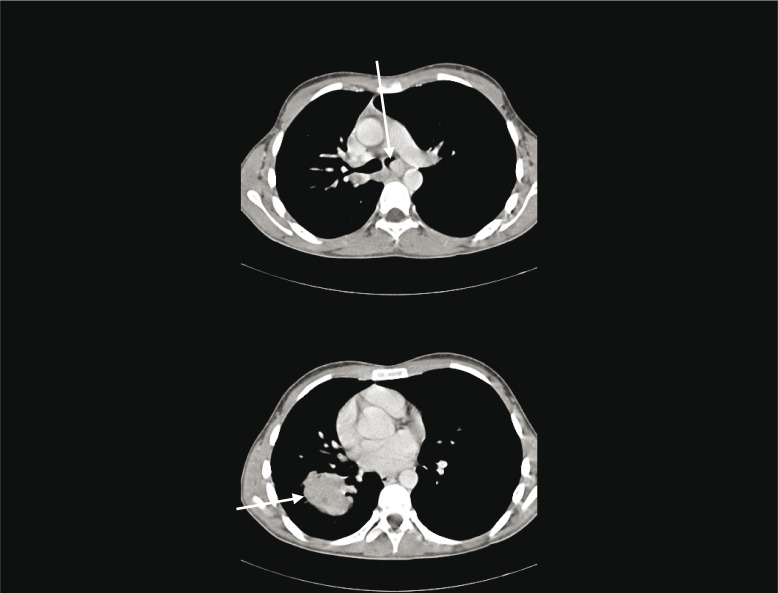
Contrast-enhanced chest CT at diagnosis. Arrows show tumour mass in left main bronchus (upper image) and in right lower lobe (lower image).
